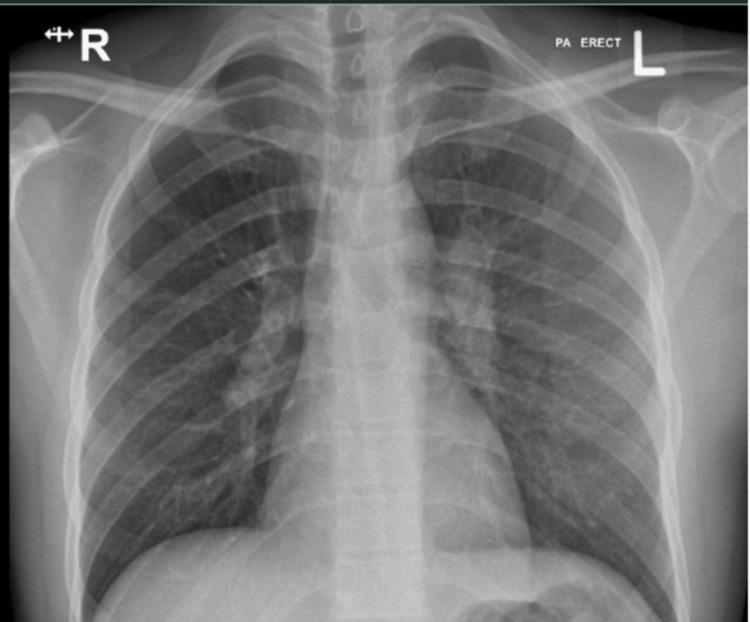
Chest X-ray showing bilateral hilar fullness
